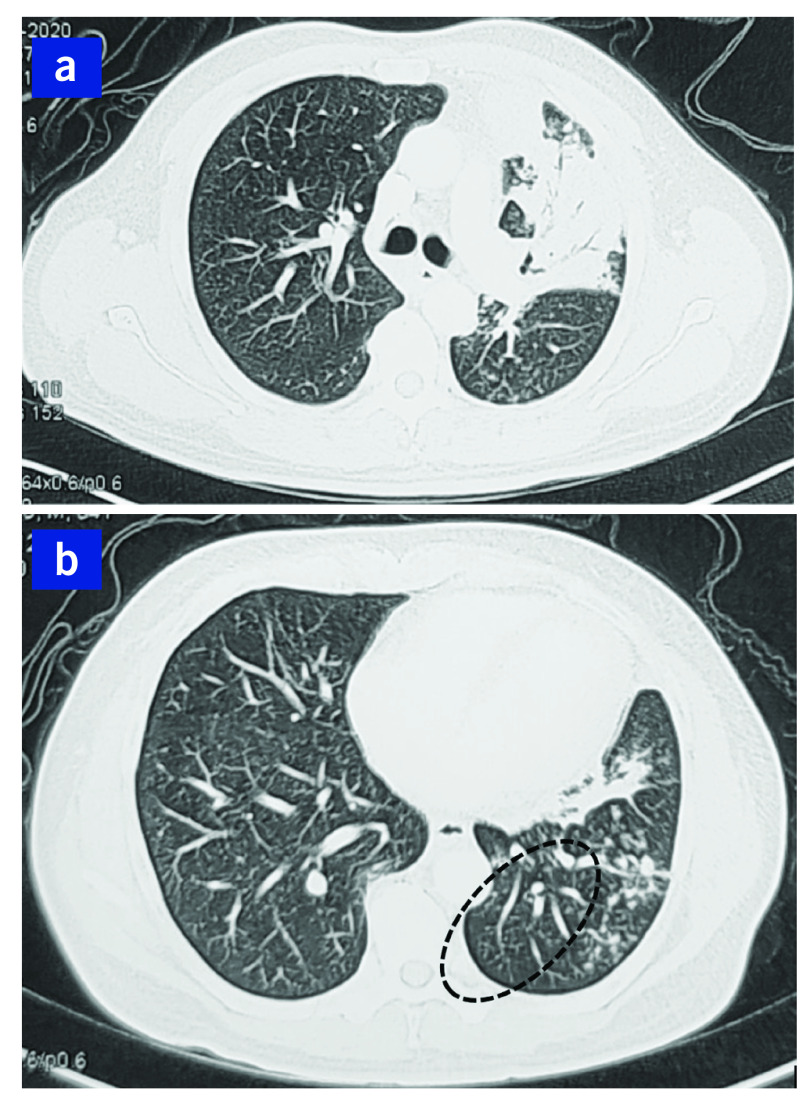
Axial computed tomography of a 64-yearold man showing (a) consolidation of the left upper lobe with volume loss and (b) the pulmonary tree-in-bud sign associated with tuberculosis affecting the peripheral pulmonary parenchyma (dotted oval)
